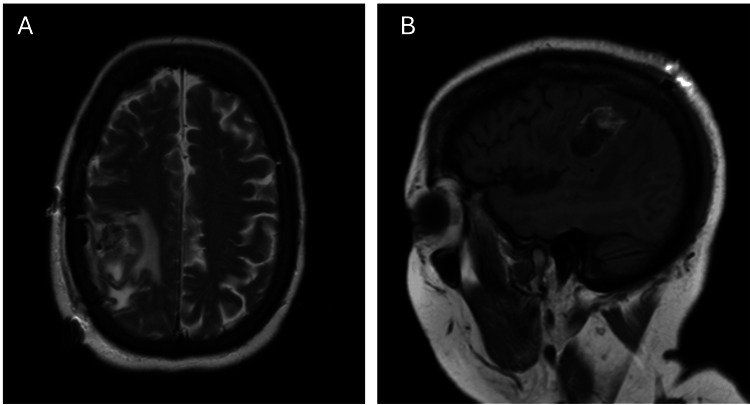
Postoperative Imaging A) Axial and B) Sagittal view of patient postoperative MRI. Gross total removal of the tumor was confirmed.
